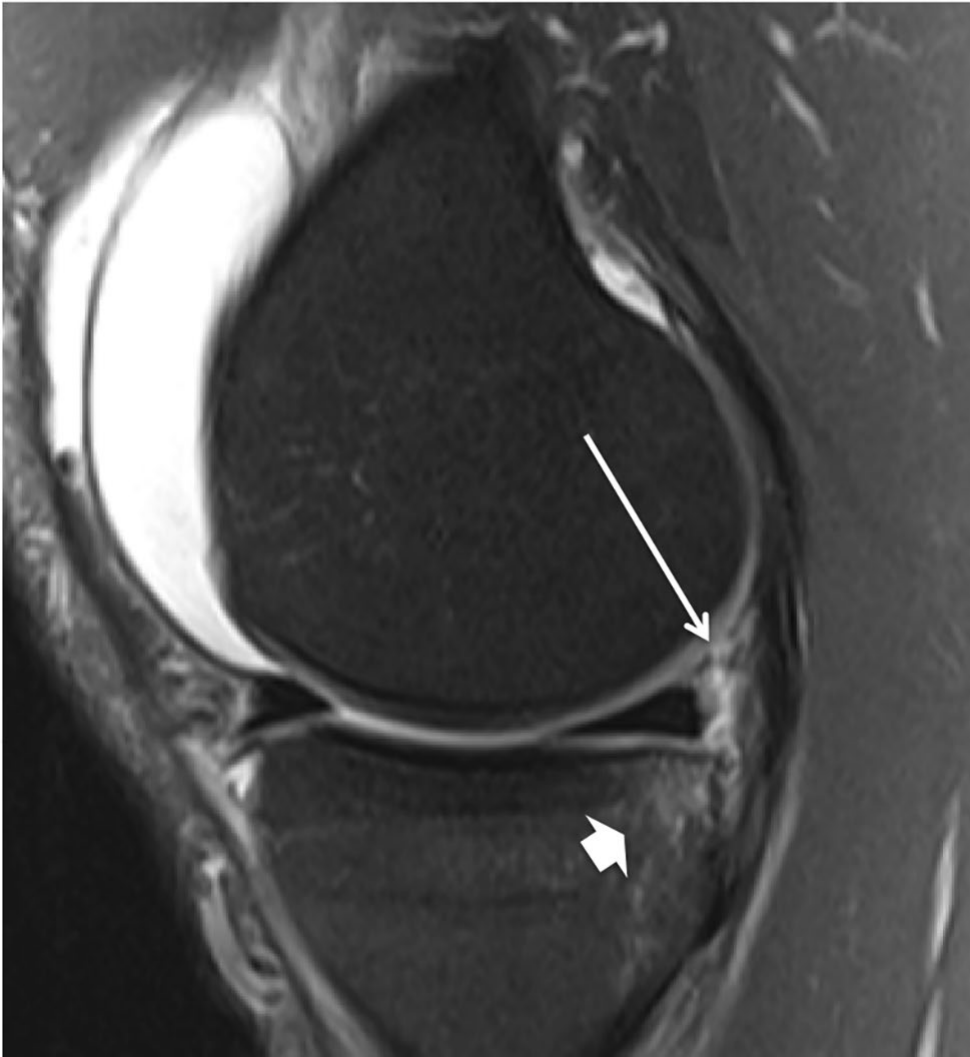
Sagittal fat-suppressed proton density weighted MRI shows a separation of the posteromedial capsule and the posterior horn of the medial meniscus (ramp lesion, long →) and bone oedema at the posterior medial tibial plateau (thick →)
